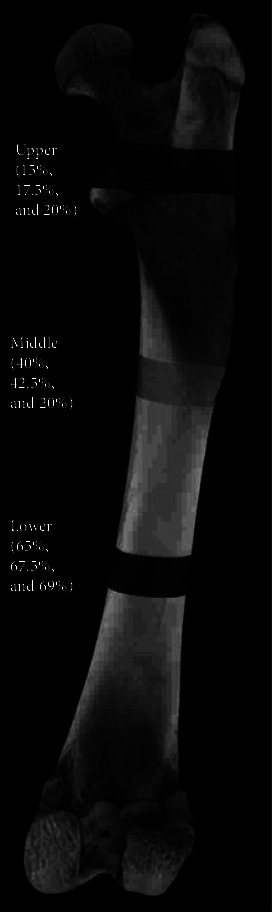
In microcomputed tomography (*μ*CT) analysis, the femora bone was divided into a planar slice and upper, middle, and lower volumes of interest (VOI).
